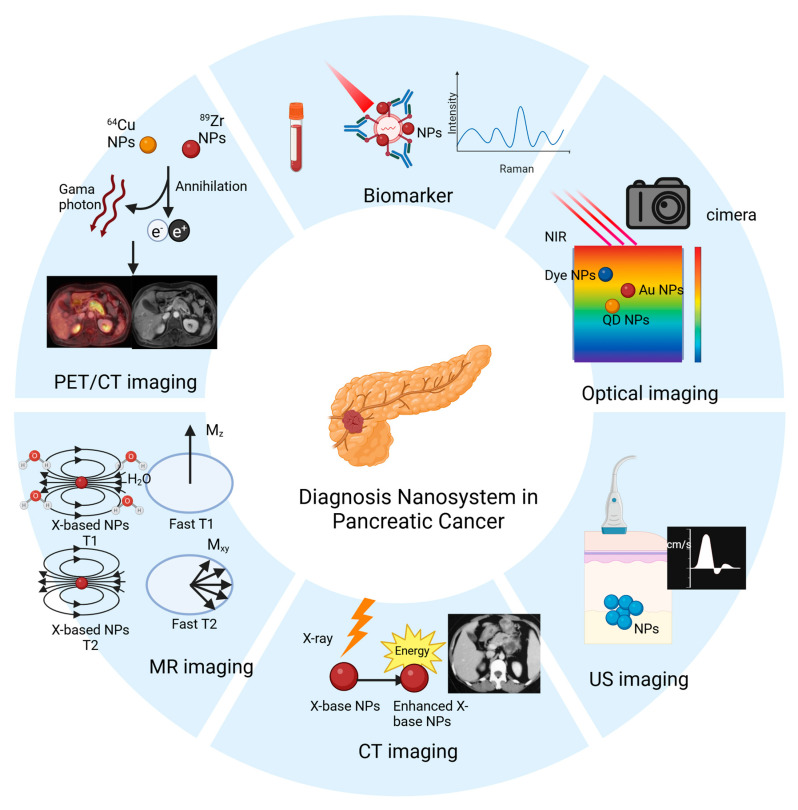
Applications of nanomaterials in the diagnosis of PDAC, including nanomaterial-based in vitro detection techniques, and nanomaterial-enabled in vivo imaging techniques (e.g., optical imaging, ultrasound (US) imaging, CT imaging, MR imaging, and PET/CT imaging).
